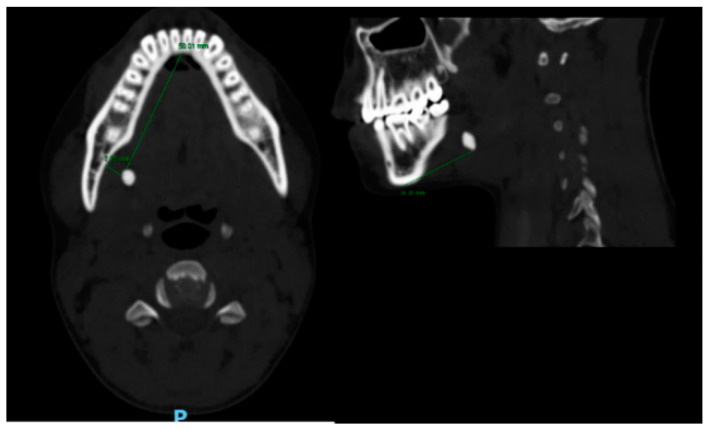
Computed Tomography (CT) of a right submandibular gland sialolith, The stone’s distance from the lingual border of the mandible (7.25 mm) and the anterior midline of the mandible (50.01 mm) (left), The stone’s distance from the inferior border of the mandible (34.26 mm) (right).
